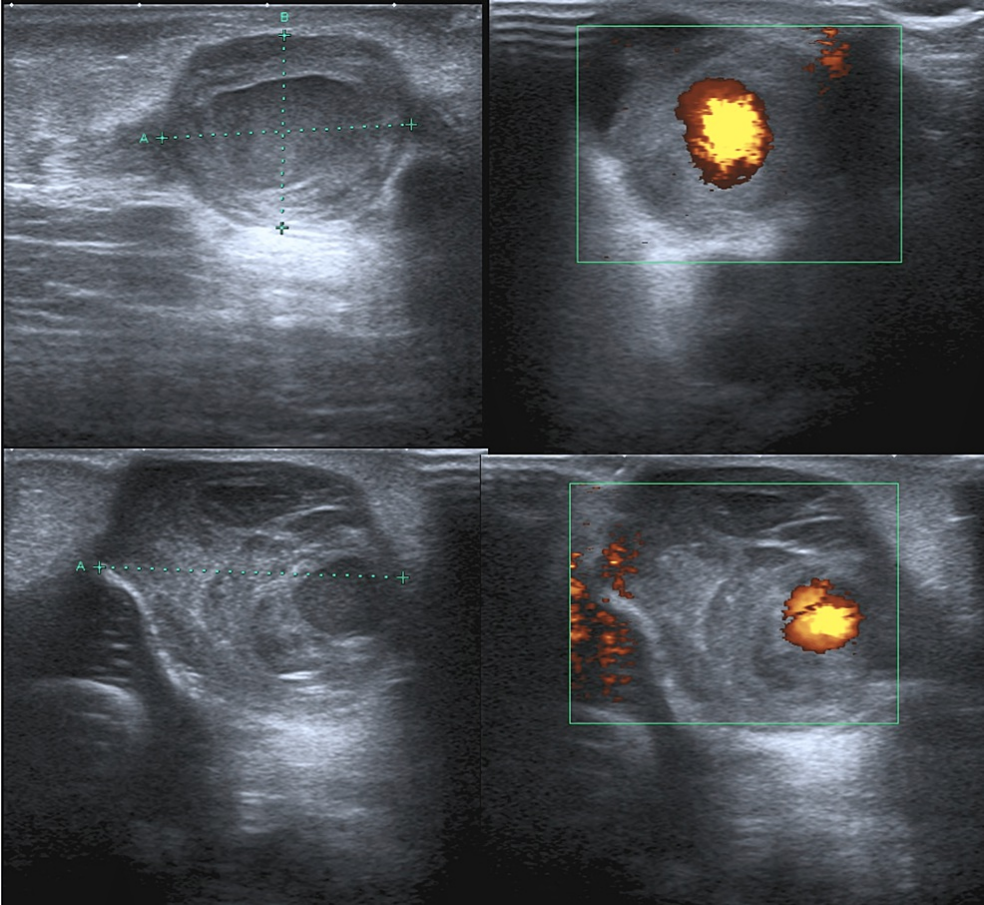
Colour Doppler ultrasound scan showing bilateral pseudoaneurysms
